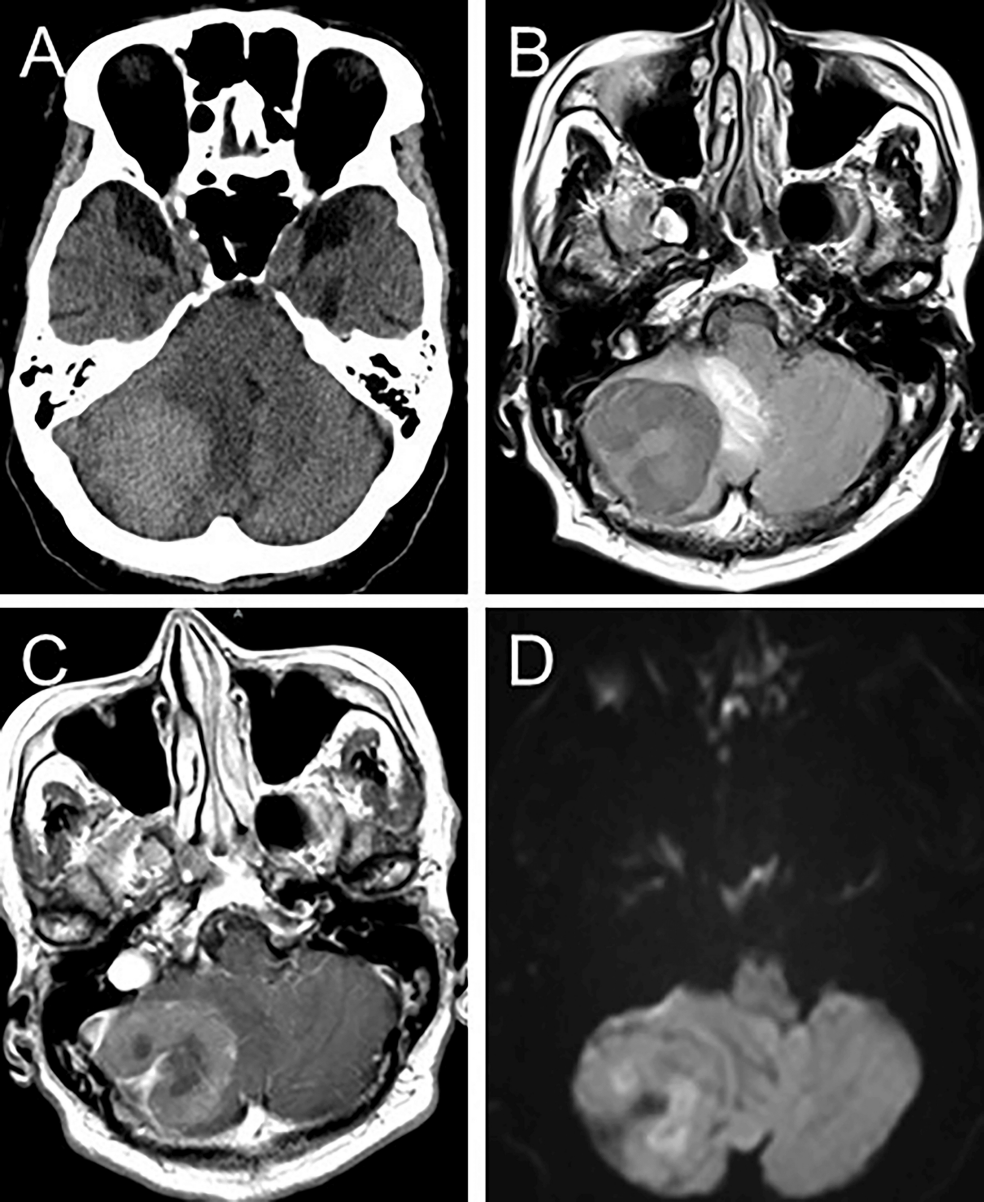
Preoperative CT and MRI (A) Preoperative axial CT reveals a hyperdense mass lesion in the right posterior fossa. (B) Axial T2-weighted MRI reveals a well-defined extra-axial hypointense and (C) T1-weighted MRI with gadolinium enhancement reveals a non-uniform enhancement of the mass in the right posterior fossa. (D) Diffusion-weighted imaging shows an isointensity mass lesion in the right posterior fossa. CT: computed tomography; MRI: magnetic resonance imaging
